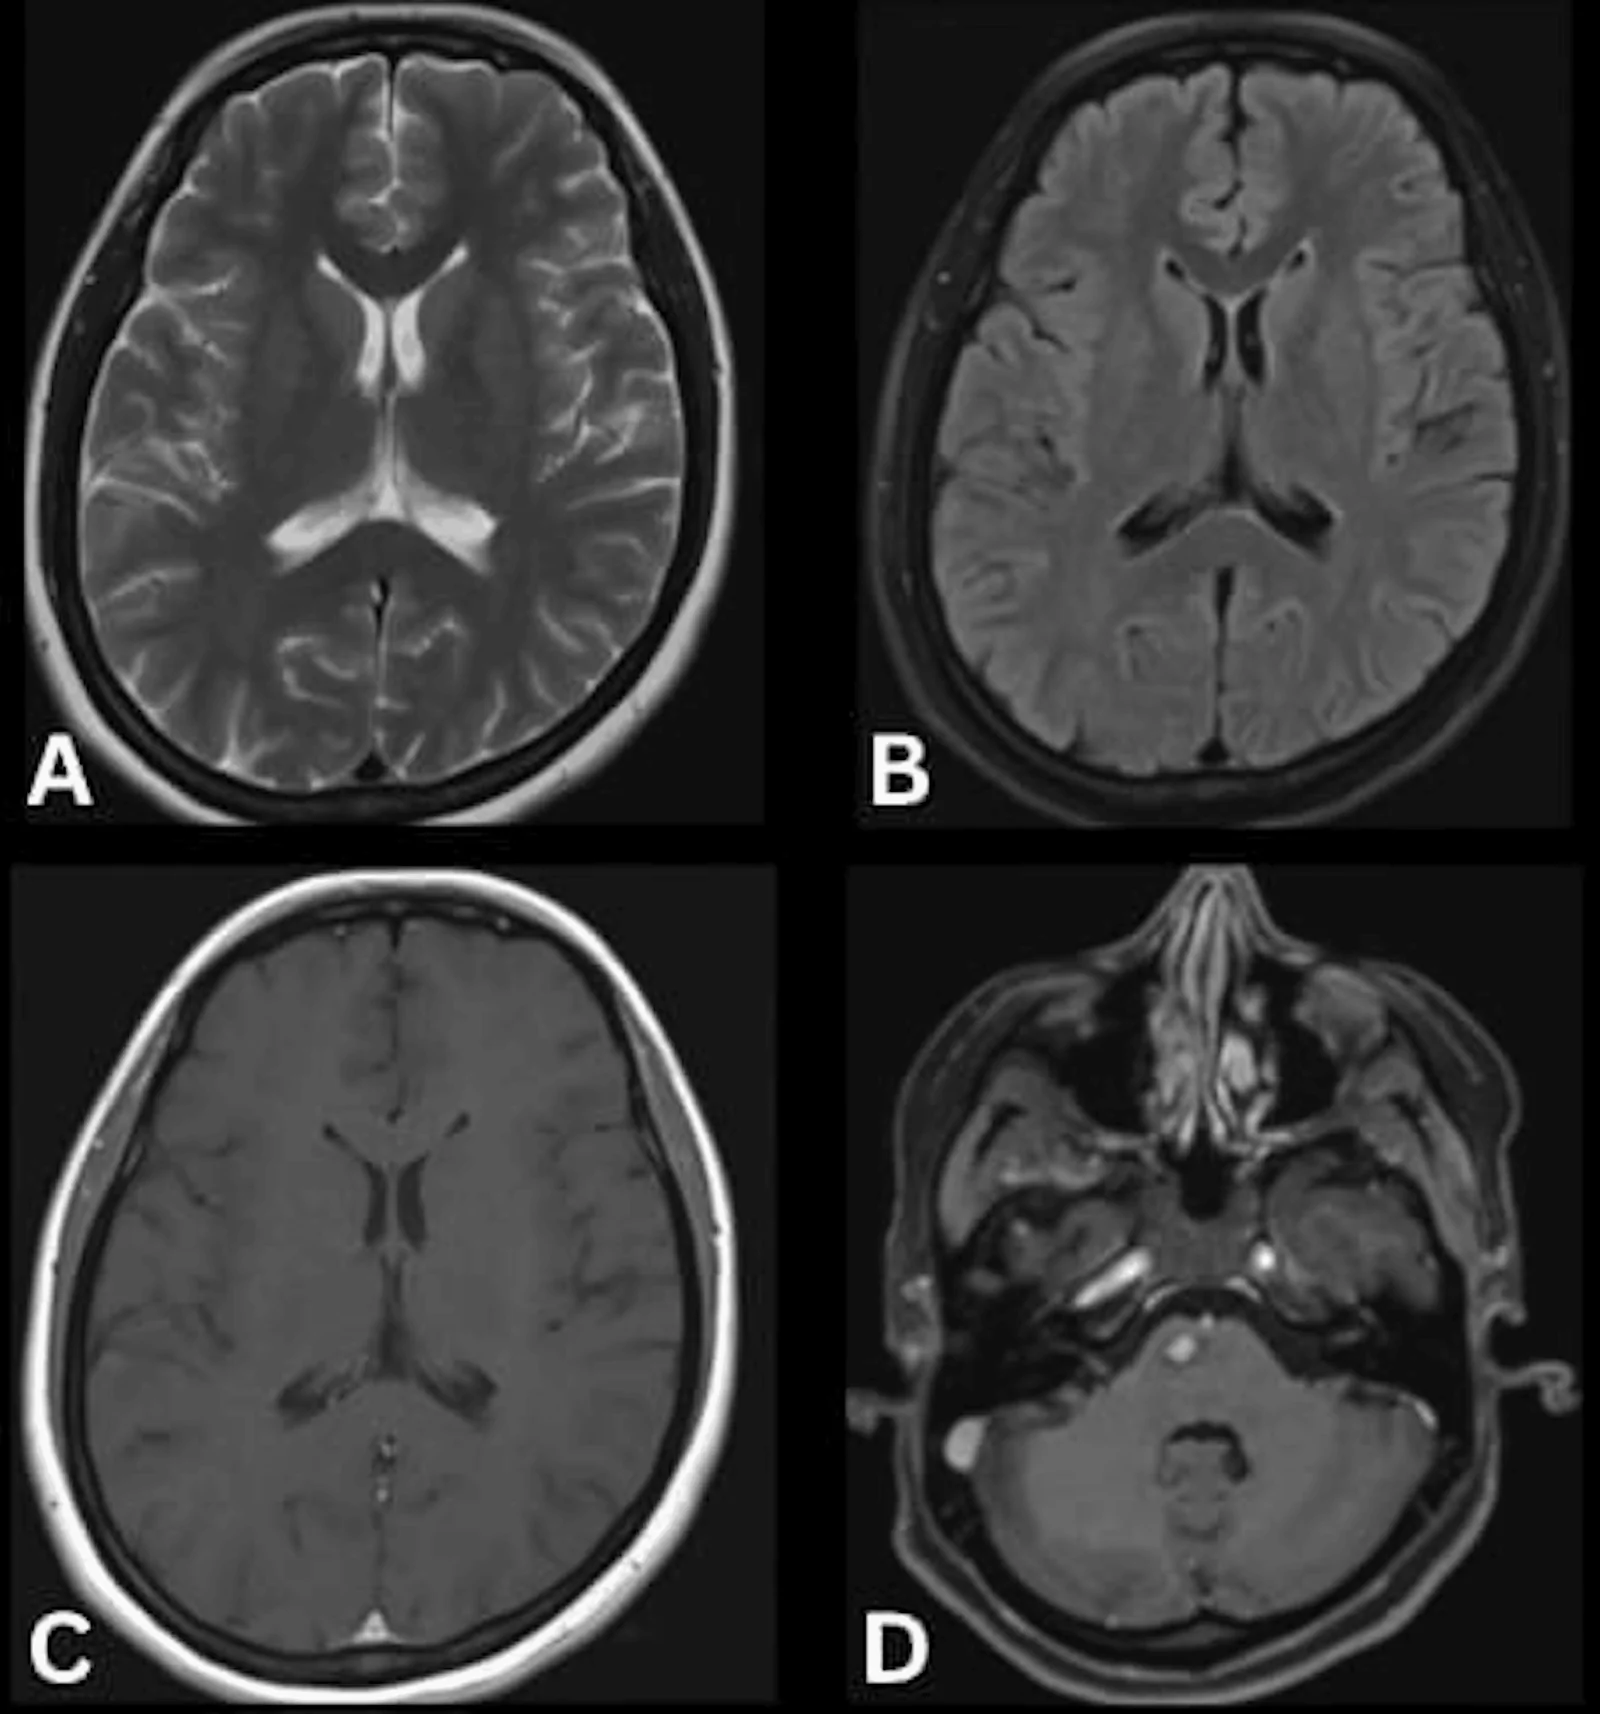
Post-treatment contrast-enhanced MRI of the brain. (A) Axial T2-weighted, (B) axial FLAIR, and (C) and (D) axial post-contrast T1-weighted images demonstrate a marked reduction in the number and size of the bilateral cerebral enhancing lesions, with significantly decreased surrounding vasogenic edema. The enhancing lesion in the right pons has also markedly decreased in size. No ventriculomegaly or midline shift is identified. FLAIR: fluid-attenuated inversion recovery.
